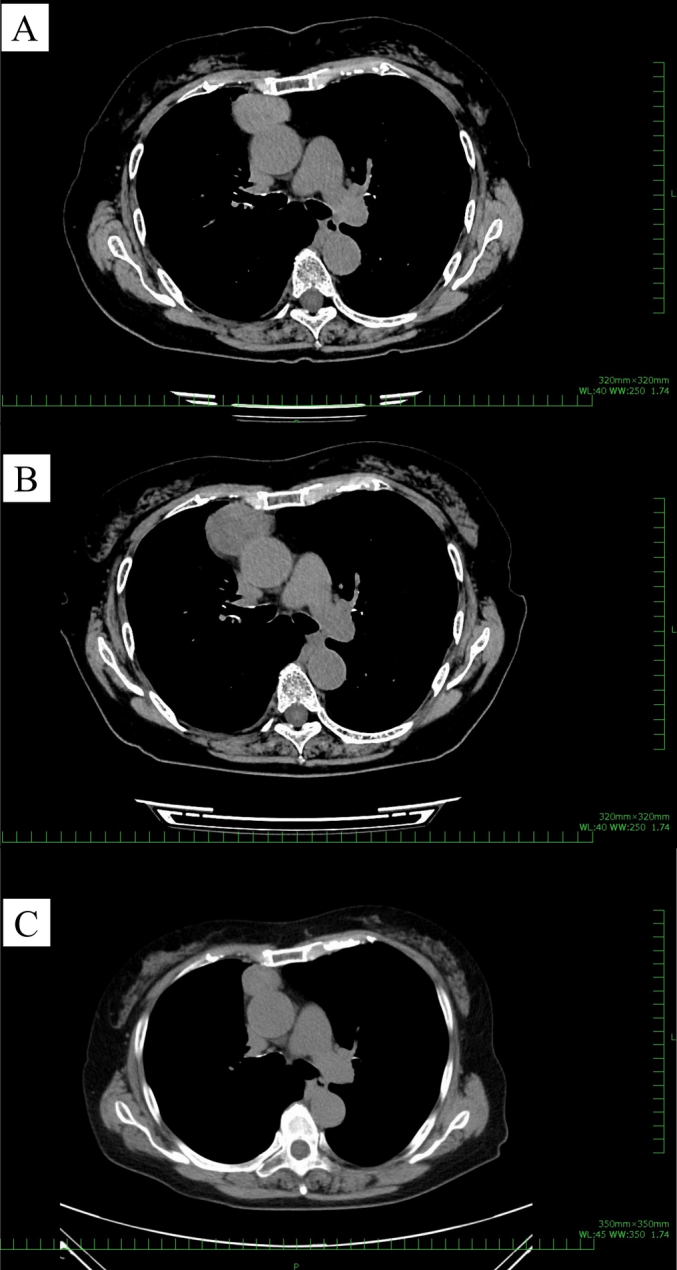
(A) Chest computed tomography (CT) showing a 41 × 32 mm anterior mediastinal tumor. (B) The anterior mediastinal tumor has slightly increased in size to 43 × 42 mm after 6 months. (C) Chest CT one day before the operation revealing that the tumor has rapidly shrunk in just one month, to 26 × 23 mm.
